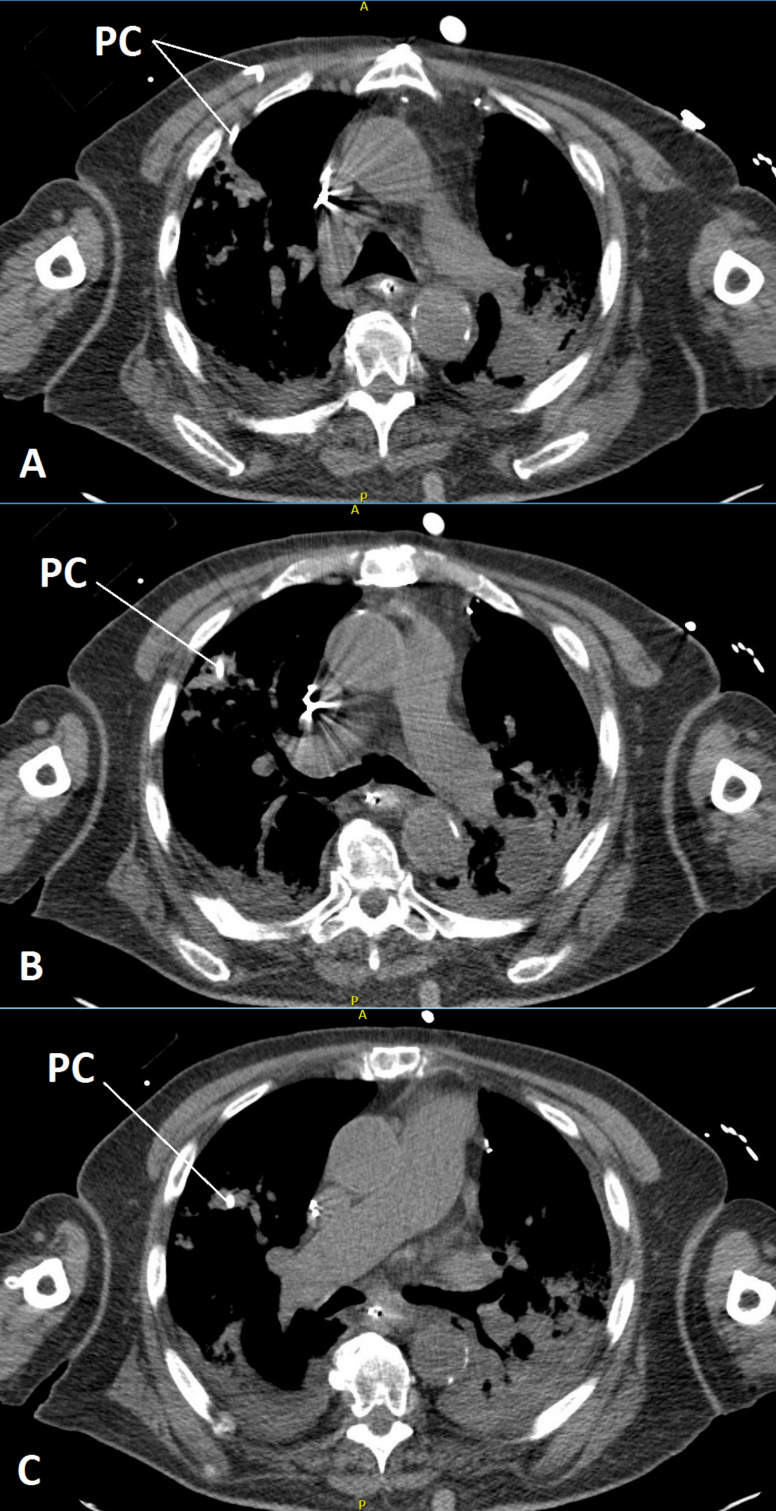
Series of computed tomography scanning showing a pigtail catheter going into the lung parenchyma and the surrounding hemorrhage/consolidation Three slices of computed tomography scans shown by A, B, and C (labeled in the lower-left corner of each scan). Small capital A and P on the top and bottom of each scan: A: anterior; P: posterior PC: pigtail catheter
